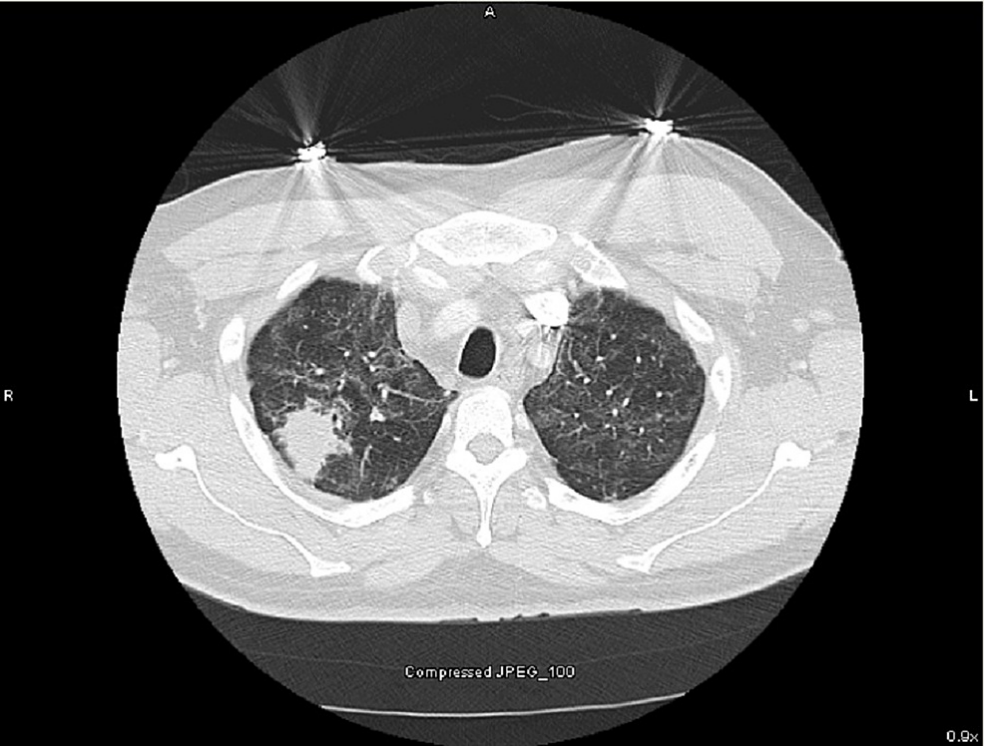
CT of the chest with right upper lobe (RUL) mass.
